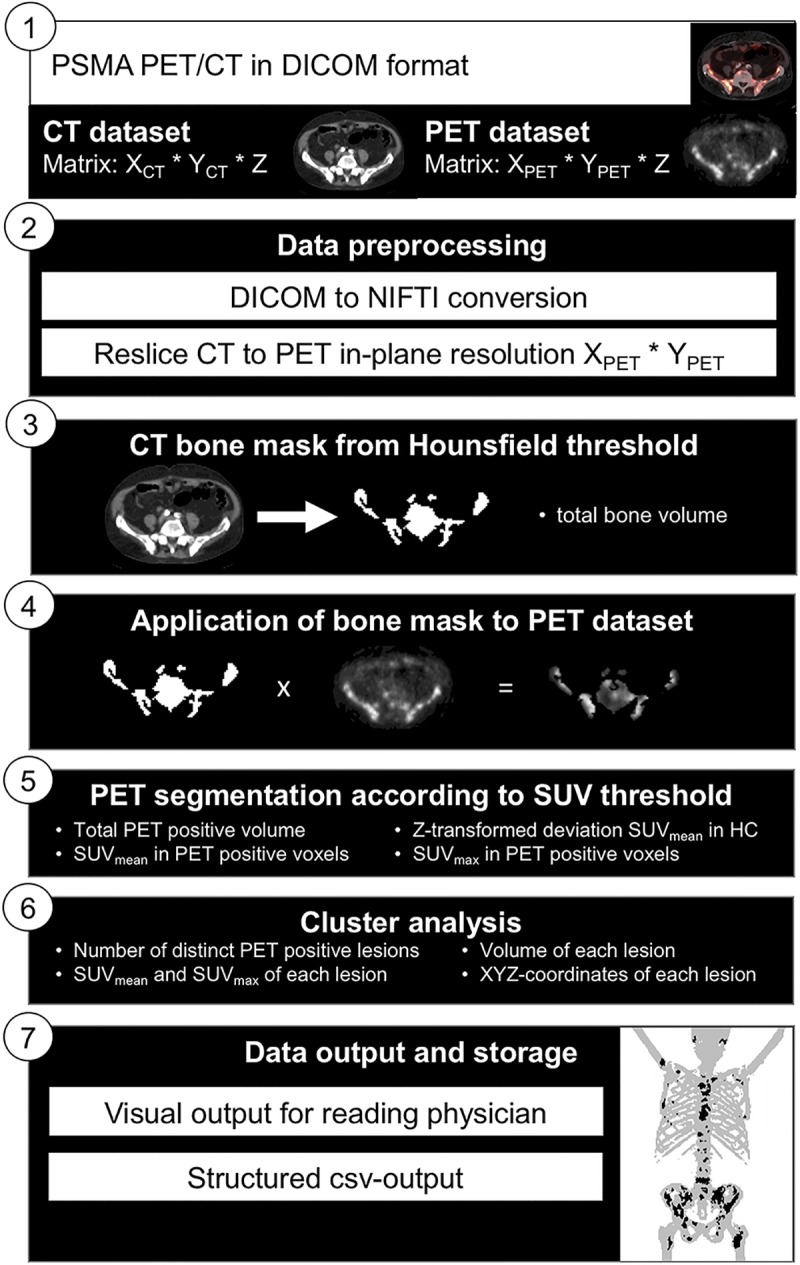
Algorithmic functionality of automated bone-VOI delineation and metastasis load quantification with EBONI in an exemplary patient with known osseous metastases of prostate cancer. csv = comma-separated values; HC = healthy controls. This figure was originally published in JNM [10]. Hammes et al. EBONI: A Tool for Automated Quantification of Bone Metastasis Load in PSMA PET/CT. J Nucl Med. 2018 Jul;59(7):1070–1075 by the Society of Nuclear Medicine and Molecular Imaging, Inc.
